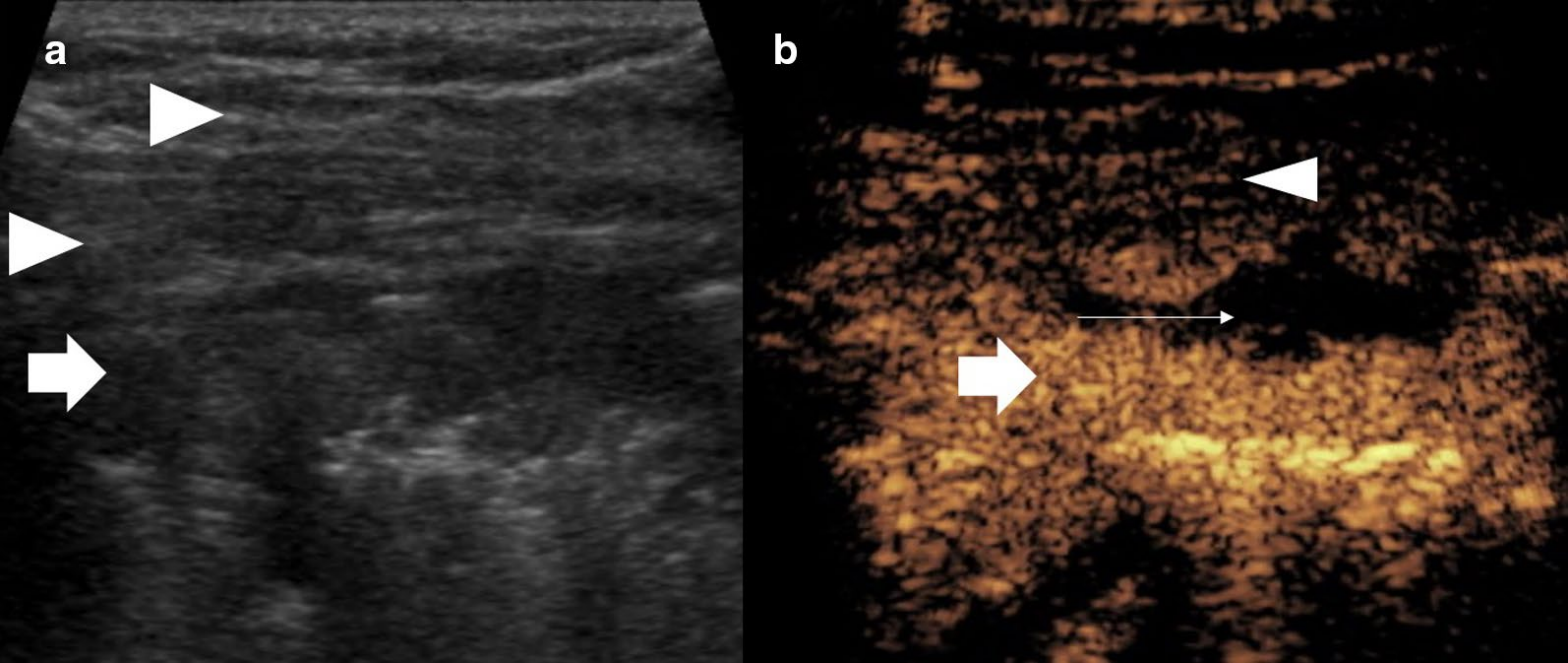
Patient with pleural mesothelioma. a B mode ultrasound shows a relatively ill-defined lesion (arrow), which is difficult to differentiate from the striated muscle at some points (arrowheads). b CEUS shows clear hyperenhancement of the lesion (arrow) defining the borders and necrotic component (thin arrow) from the straited muscle layer (arrowhead) allowing accurate targeting for an optimal biopsy sample
